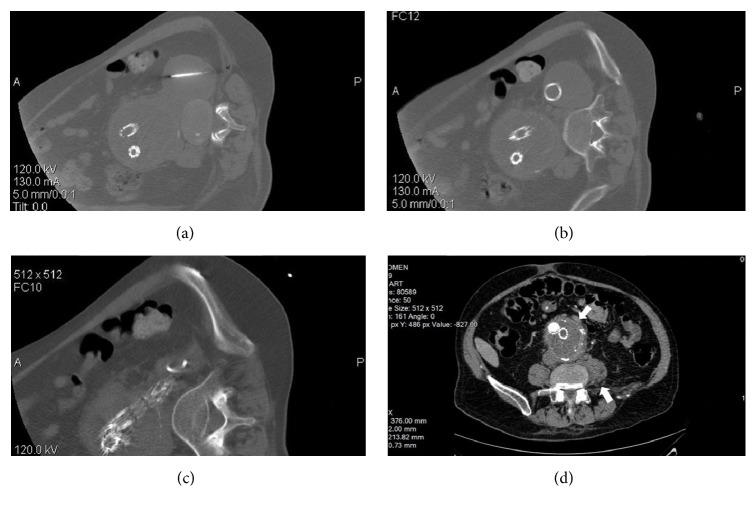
Drainage of hematoma under CT guidance: (a) guide trocar inserted from lumbar (b) pigtail in place drains the hematoma; (c) immediate shrinkage of hematoma cavity; (d) left iliopsoas muscle with almost normal shape (upward arrow) and reduction of remaining aneurysm sac (downward arrow) in a follow-up CT imaging four months later.
